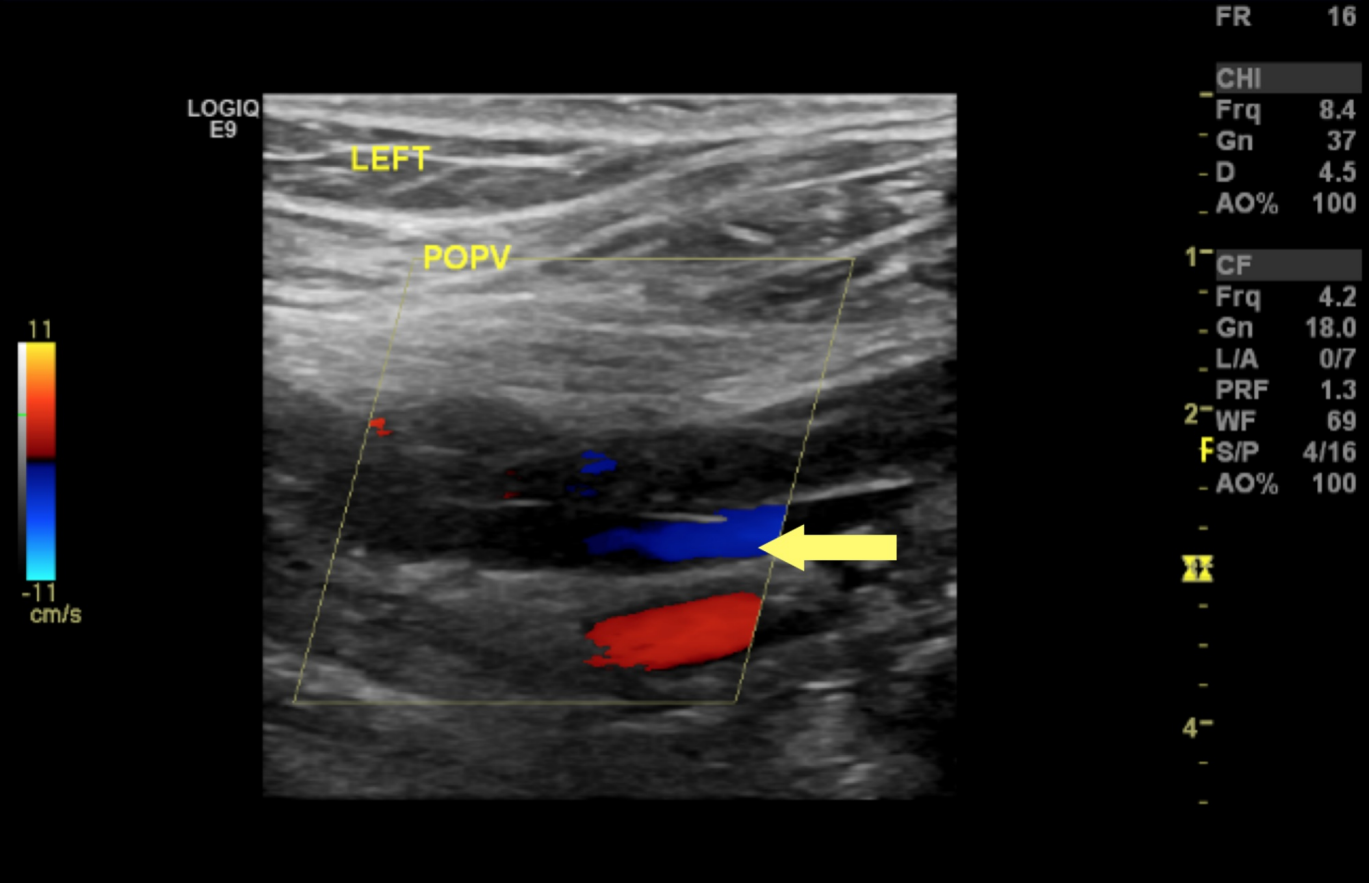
Doppler ultrasound for left popliteal vein showing partial occlusion
